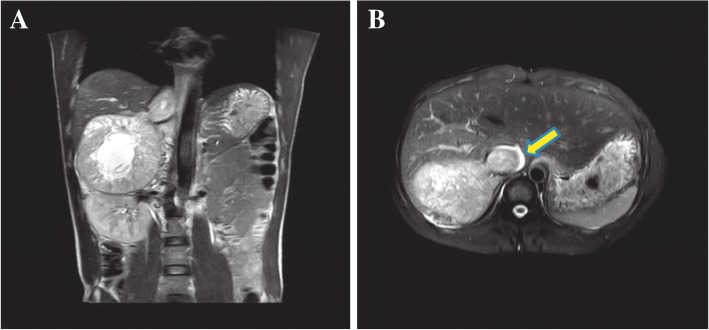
a. Magnetic resonance image of the abdomen shows the proximal part of inferior vena cava was widened on longitudinal scan. b. Magnetic resonance image of the abdomen indicated tumor thrombus in the inferior vena cava next to the large adrenal phaeochromocytoma on horizontal scan
